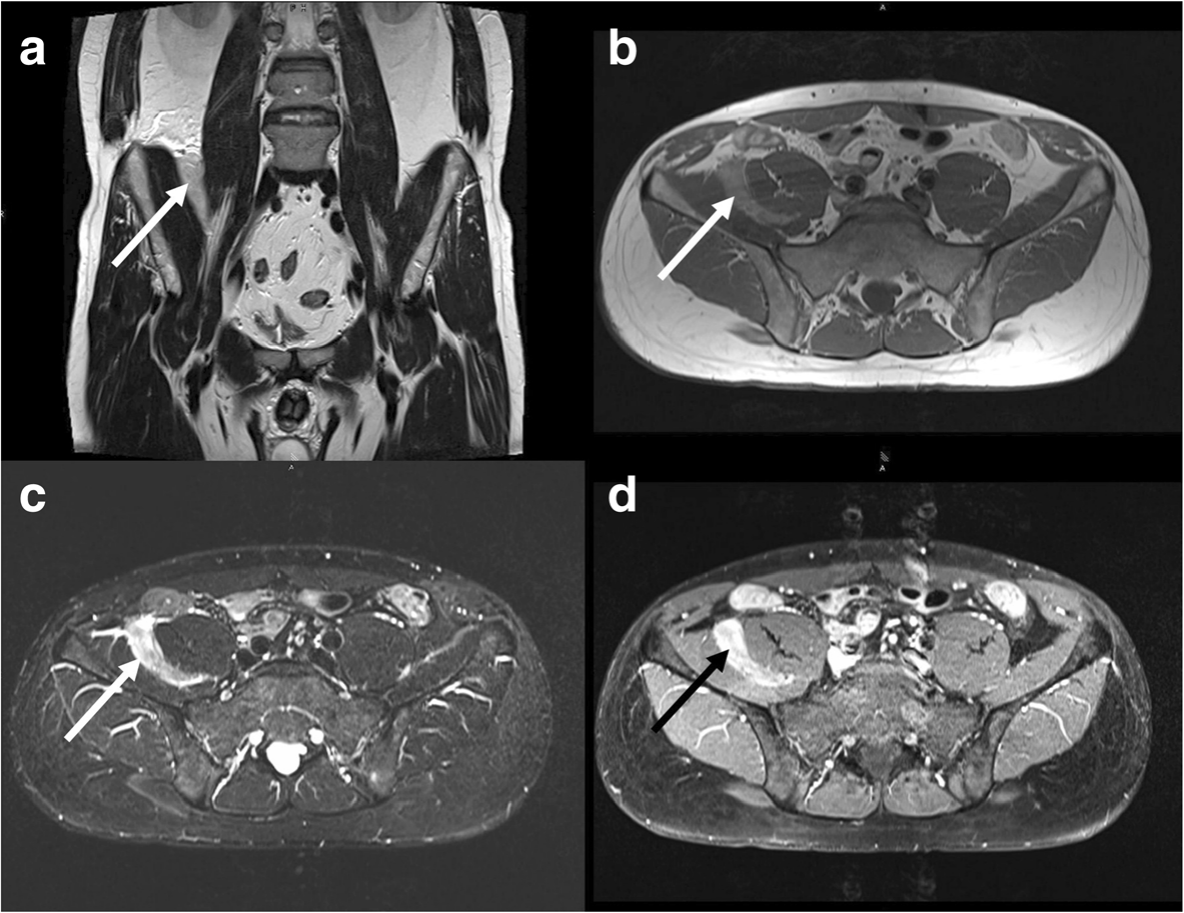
MRI of the same patient as shown in Figure
